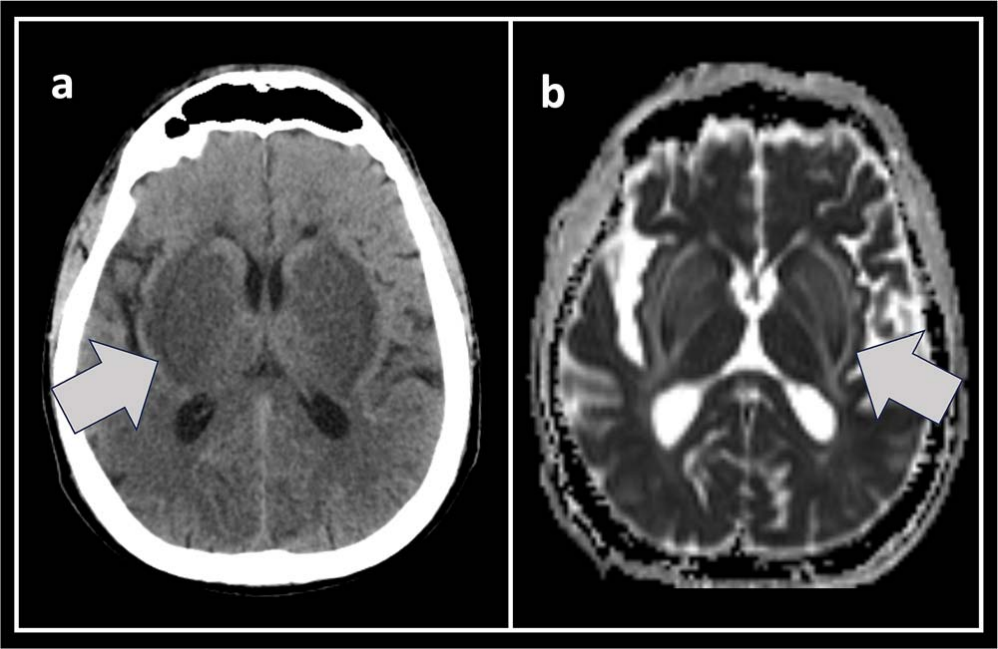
The Lentiform fork sign. The non-enhanced CT (subset a) and ADC map (subset b) with the lentiform fork sign. The arrows point to the lentiform fork. Non-enhanced CT show bilateral symmetrical basal ganglia hypodensities, bordered laterally by a black line corresponding to the external capsule. ADC map show three linear hyperintensities delineating the internal, external capsules and the medullary laminae, CT—Computed tomography, ADC—Apparent diffusion coefficient.
